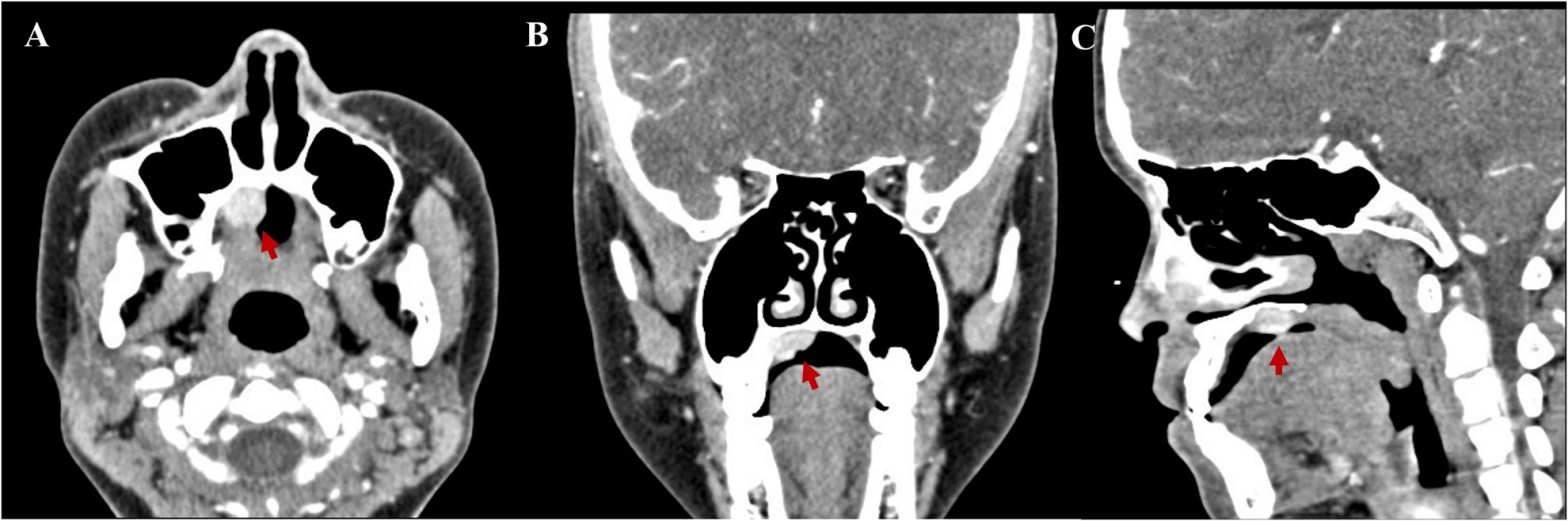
Contrast-enhanced computed tomography scans revealed a quasi-circular soft tissue mass of the right hard palate 1.5 cm × 1.2 cm × 1.0 cm in size. (A) Axial, (B) coronal, and (C) sagittal.
